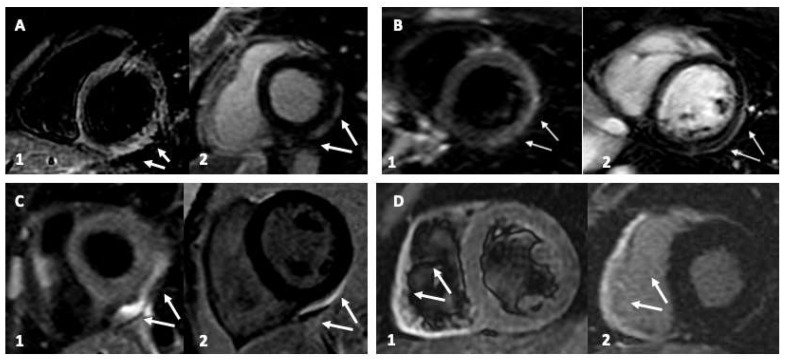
Cardiac magnetic resonance (CMR) imaging of young patients with vaccine-related myocardial involvement. Panel (A,B): Inferolateral myocardial involvement with edema and late gadolinium enhancement (LGE), with a subepicardial and pericardial distribution typical of myocarditis. Panel (C): Only pericardial involvement. Panel (D): isolated right ventricle involvement. Sub-Panel 1, triple IR (Triple-IR) FSE T2-weighted images for myocardial edema detection. Sub-Panel 2, LGE images.
